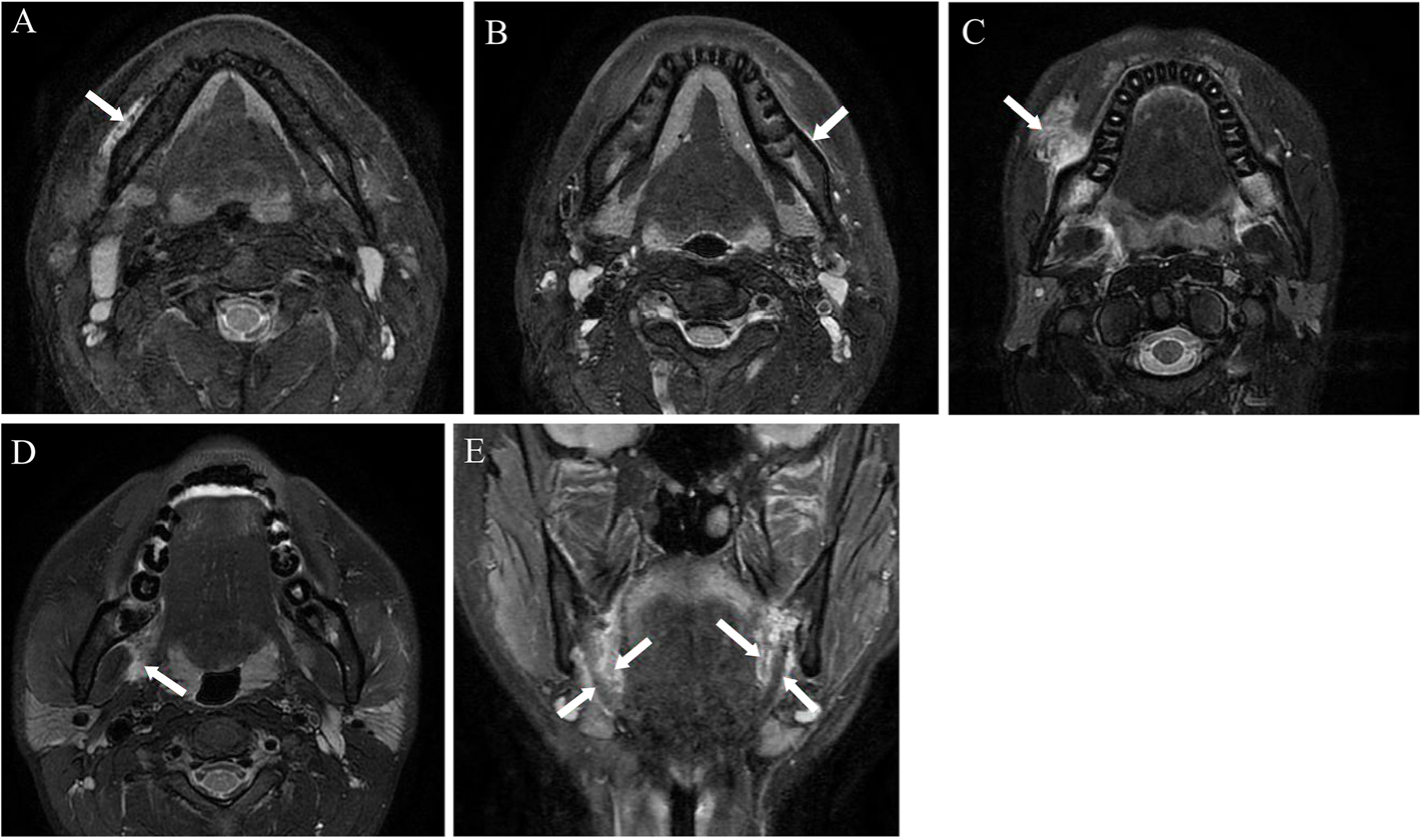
Examples of edema in the postoperative spaces on magnetic resonance imaging. a Buccinator muscle (arrow). b Supra-periosteum space (edema space observed along the outer border of the mandible, arrow). c Buccal space (arrow). d Parapharyngeal space (arrow). e Lingual space (edema space on sublingual or submandibular space, arrows)
